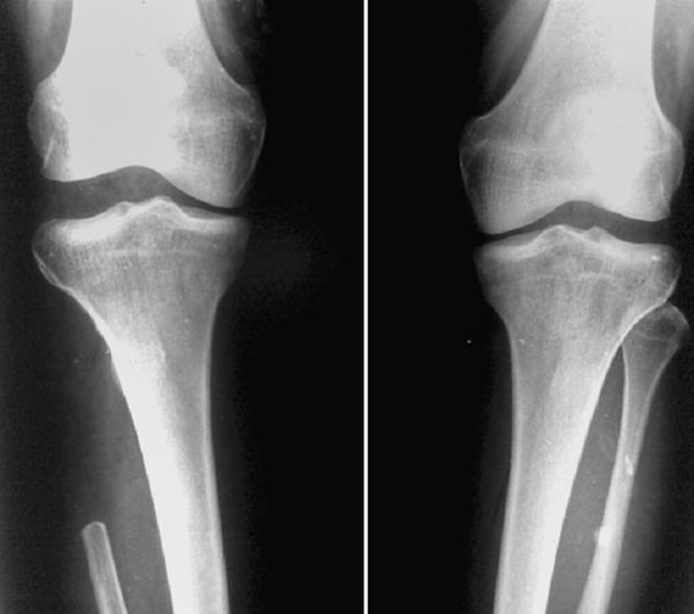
Varus stress radiograph of both knees showing a significant increase in lateral joint space after resection of the proximal fibula on the right side
